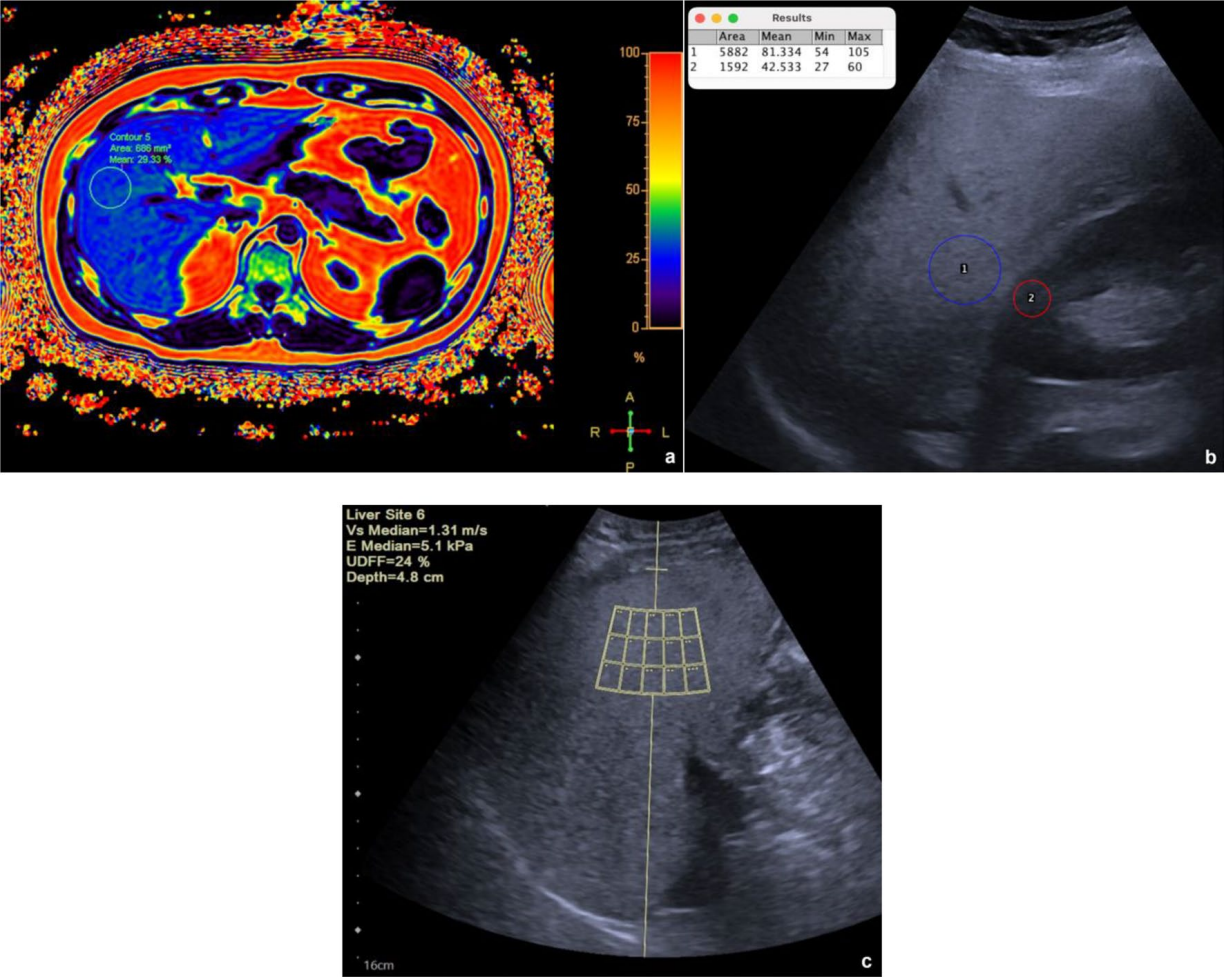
Quantification of liver fat content in a 31-year-old man with NAFLD. a Fat fraction map: a circular ROI is visible in liver segment 5; the overall median MRI-PDFF value was 25%. b B-mode US and quantification of the hepatorenal index: liver echogenicity is higher than the right kidney cortex; visualization of the diaphragm and posterior surface of the right hepatic lobe is decreased; the ratio of the mean brightness of liver (1) and kidney (2) ROIs was 1.91; c UDFF measurement: a ROI box is visible in liver segment 5; the overall median UDFF value was 24%
